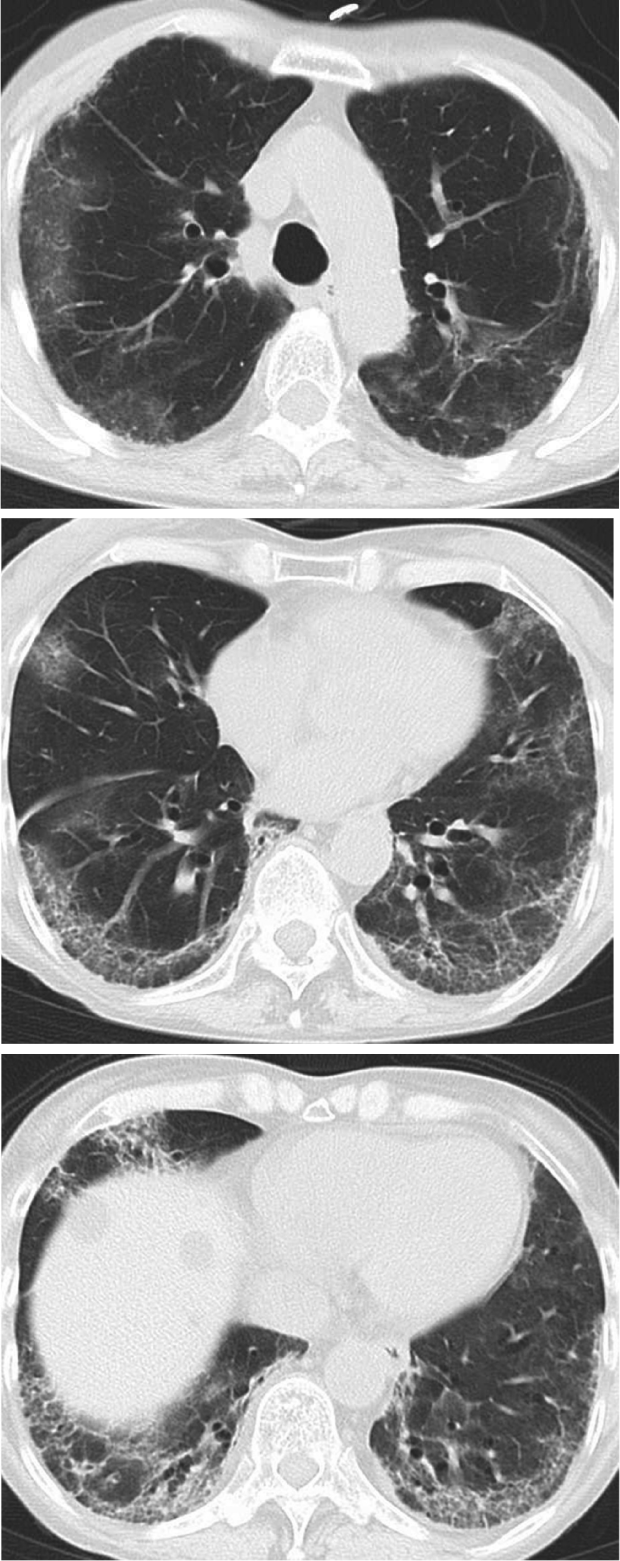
Computed tomography after 8 months from the onset.
